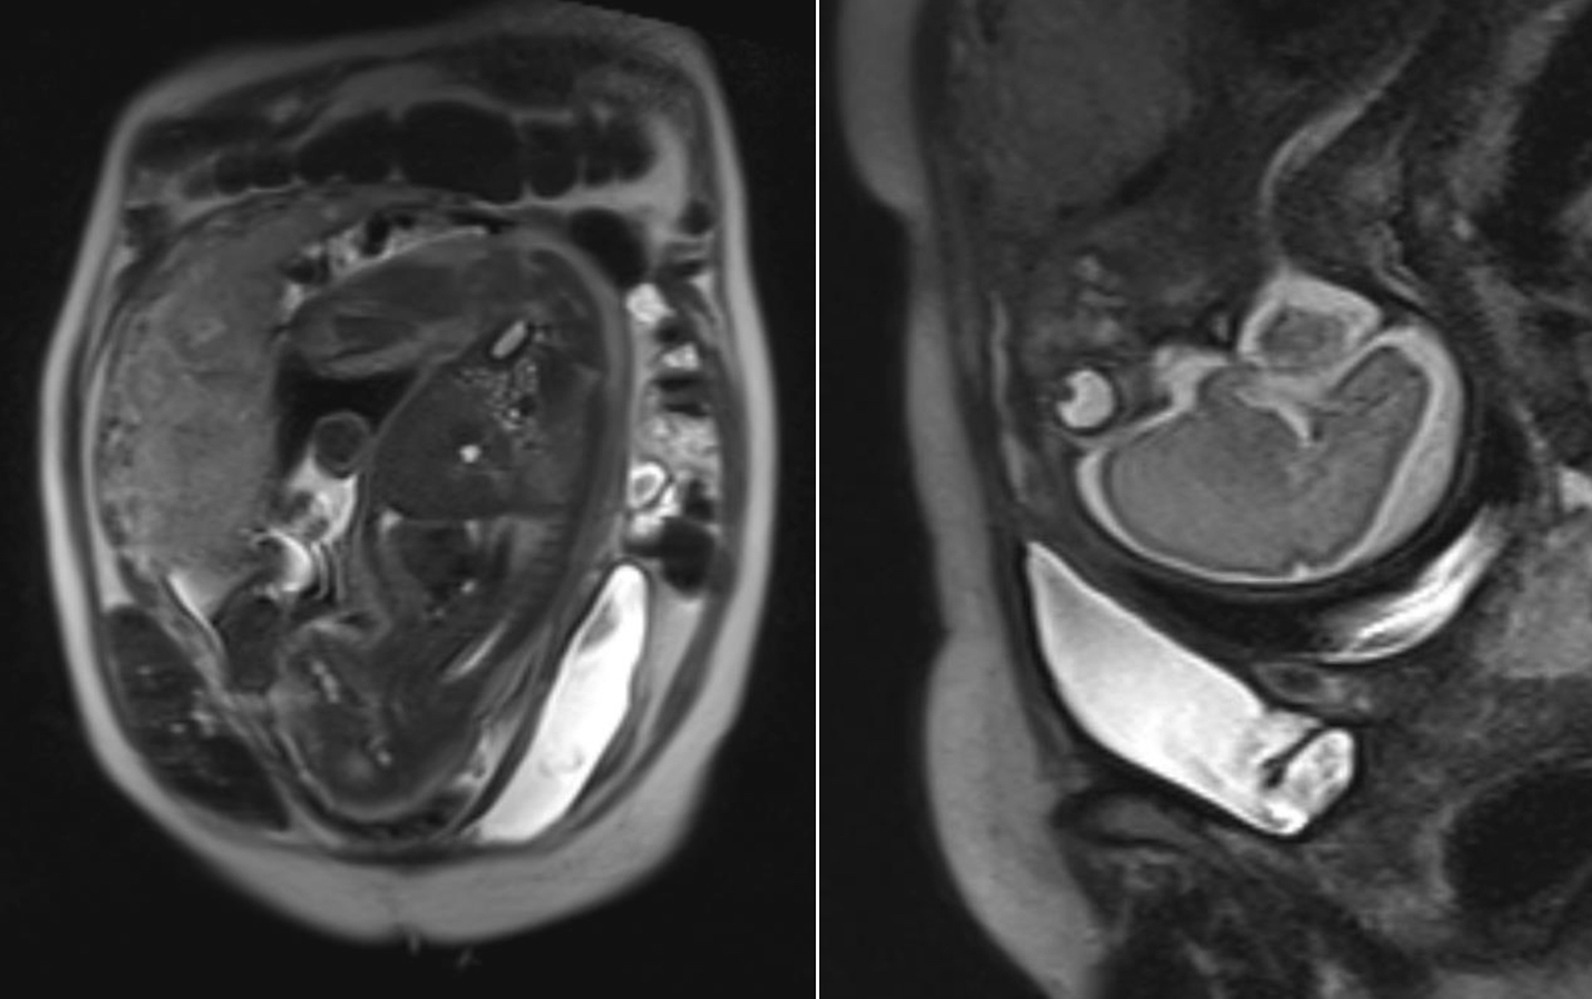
An MRI performed at 31 weeks of gestation shows anatomical relationship and dislocation of neobladder. After retrograde voiding, the neobladder appears to be dislocated in left antero-lateral position to the uterus. No MRI signs of tissue adherence between neobladder and uterus wall are observed
